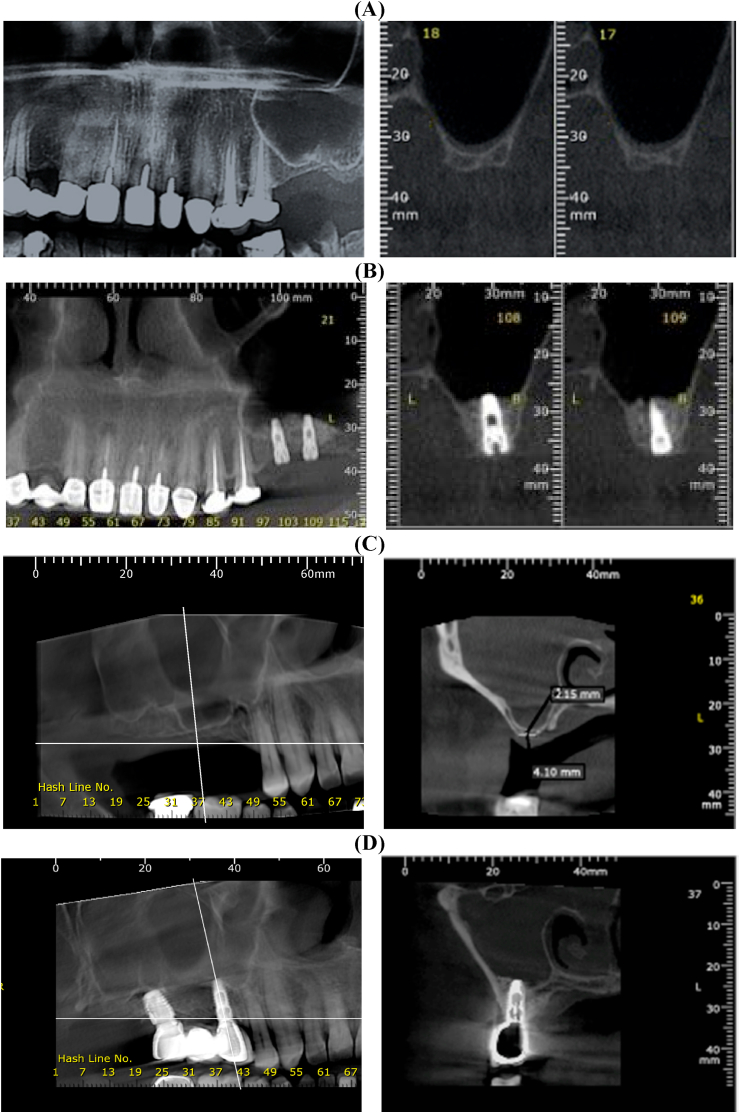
Cross-section on CBCT, (A) Preoperative showing normal sinus with low vertical bone height, (B) 6-month post-operative showing increase in bone height following osseointegrated implant and healing graft, and (C) pre-operative cross-section on CBCT showing chronic sinusitis with low vertical bone height. (D) 6-month post-operative cross-section on CBCT showing increased vertical bone height after osseointegrated implant and healing graft.
